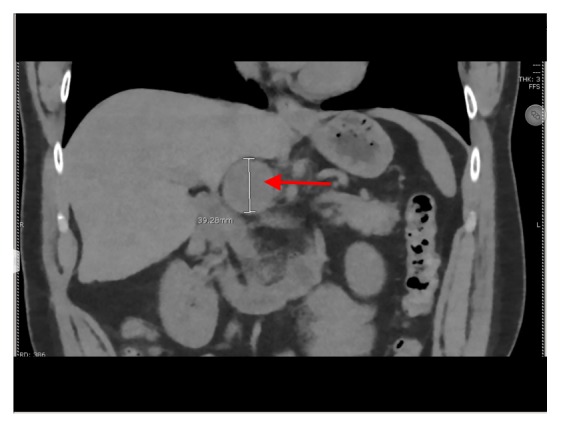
CT abdomen with porta hepatis paraganglioma.
